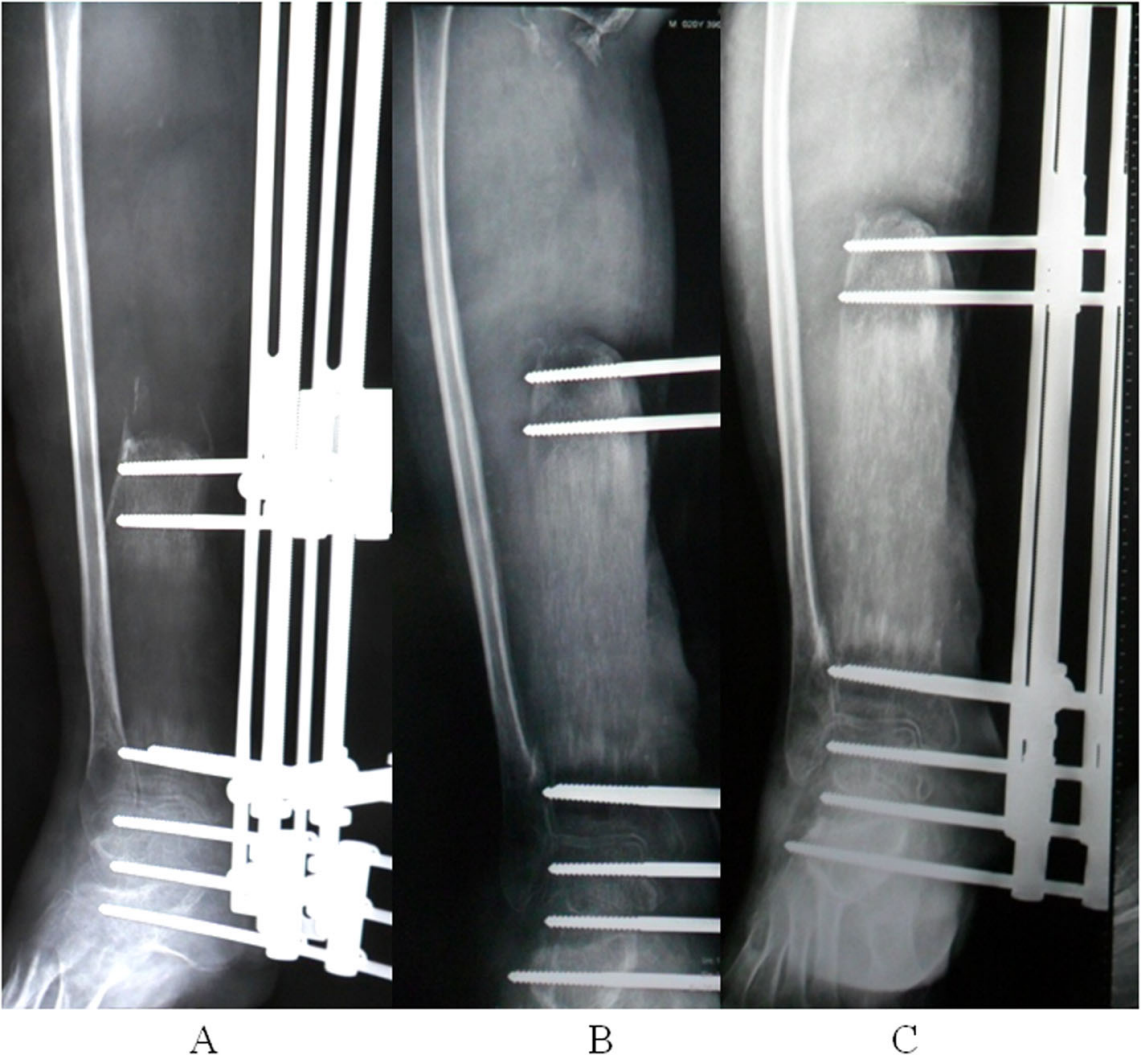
X-ray performed (a) 2, (b) 4 and (c) 6 months after corticotomy demonstrating bone regeneration
